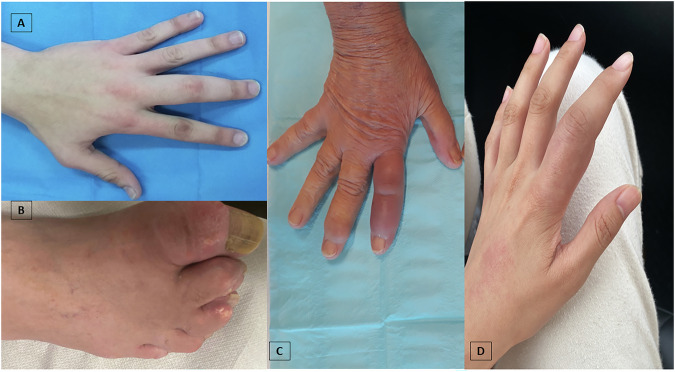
Dactylitis related to rheumatologic disorders. A Inflammatory dactylitis in a patient with psoriasis involving the elbows and knees, presenting with pain and functional limitation of the third finger of the left hand. The dactylitis showed a rapid and marked response to ultrasound-guided corticosteroid injection into the flexor tendon sheath. B Chronic dactylitis in a 70-year-old patient with known gouty arthritis, presenting with multiple episodes of diffuse swelling of the first toe of the right foot. The patient was not receiving therapy due to poor compliance. The clinical picture was consistent with tophaceous deposition, also involving other digits. C Acute dactylitis in an 82-year-old patient with known calcium pyrophosphate deposition (CPPD) disease arthritis, presenting with first episode of diffuse swelling of the second finger of the right hand. Other causes were excluded. The clinical picture was consistent with crystal-induced inflammatory dactylitis and showed a good response to a course of colchicine. D Dactylitis in a middle-aged woman with sarcoidosis, presenting with diffuse swelling and pain of a finger, associated with respiratory symptoms (dry cough and dyspnea) and peripheral arthritis, with excellent response to anti–TNF-α therapy.
